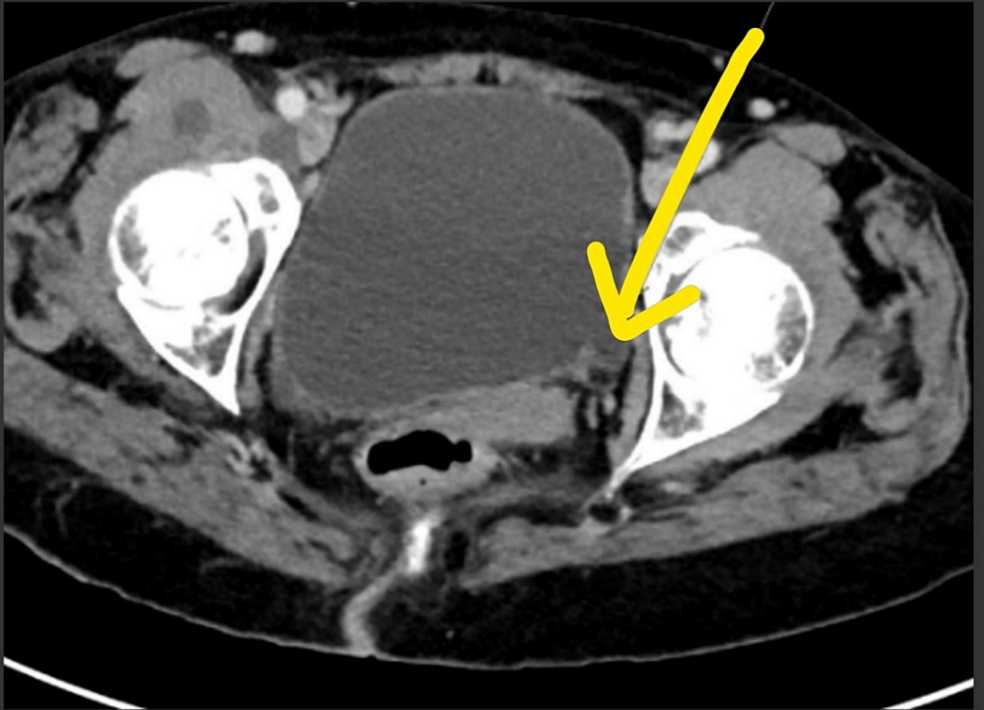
Another left-sided bladder diverticula; yellow arrow inserted to show outpouching of the bladder wall mucosa. CT abdomen and pelvis
